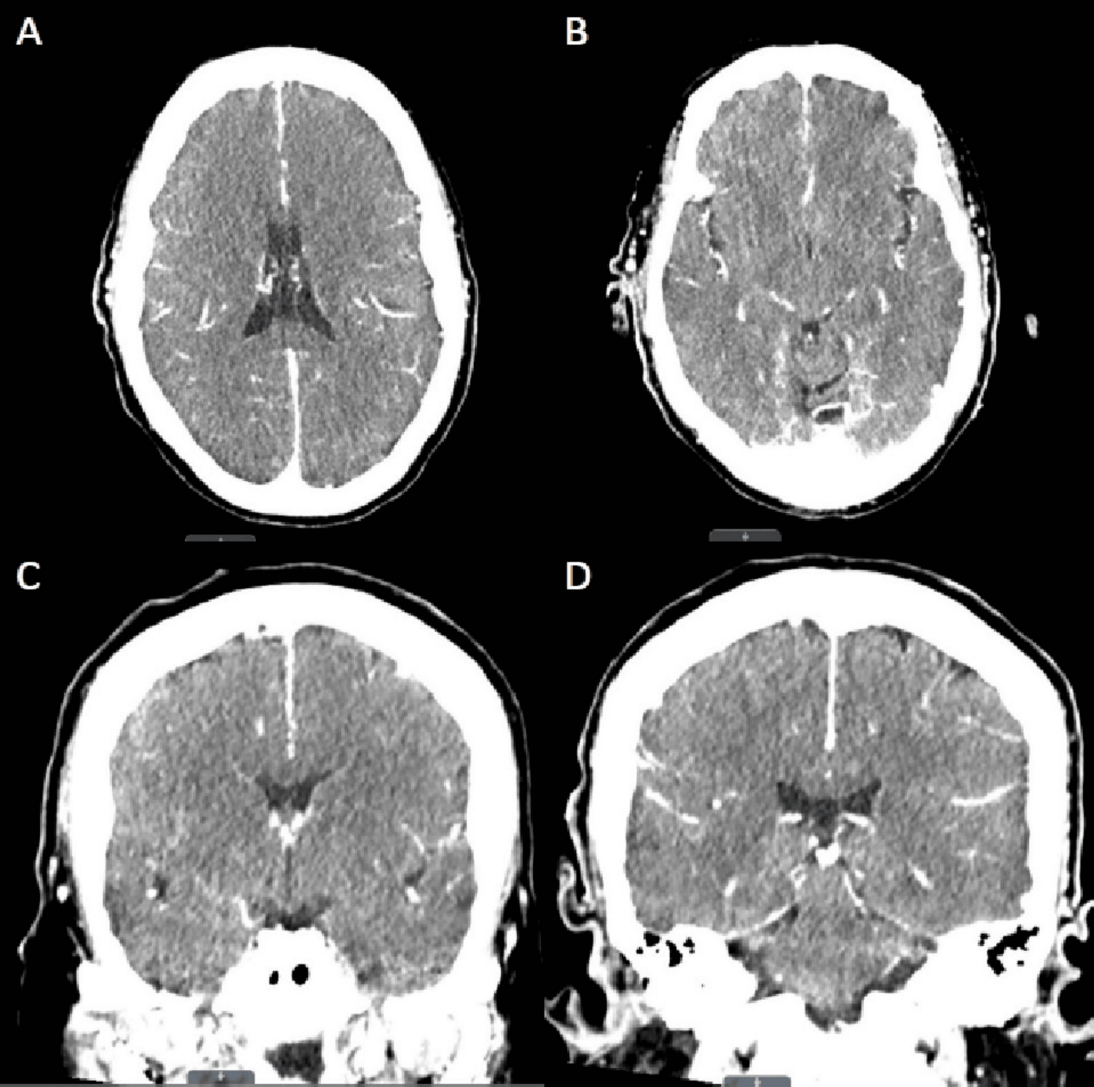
Contrast-enhanced CT of the head showing mild cerebral edema. (A) Axial image at the level of the lateral ventricles; (B) Axial image at the level of the aqueduct of Sylvius; (C) Coronal image at the level of the optic tract; (D) Coronal image at the level of the superior colliculus.
